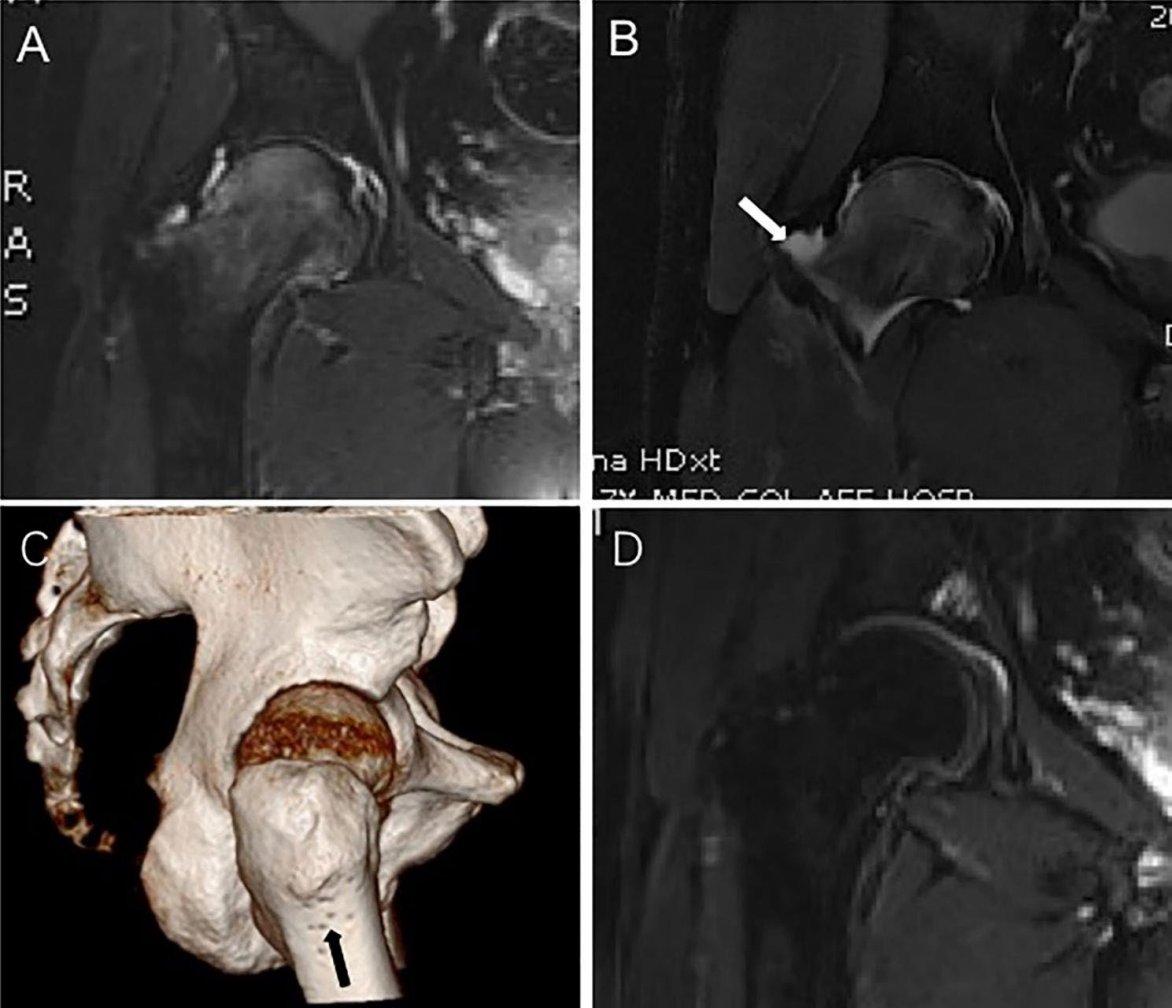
A Preoperative MRI T2-weighted coronal image of right hip demonstrated a bone marrow edema of femoral head and neck. B MRI T2-weighted coronal image of right hip showed an effusion pattern of the hip cavity (white arrow). C Postoperative 3D imaging showed the tracks of multiple drilling decompression at lateral side of proximal femur (black arrow). D Postoperative MRI T2-weighted coronal image of right hip demonstrated disappearance of bone marrow edema of femoral head and neck
